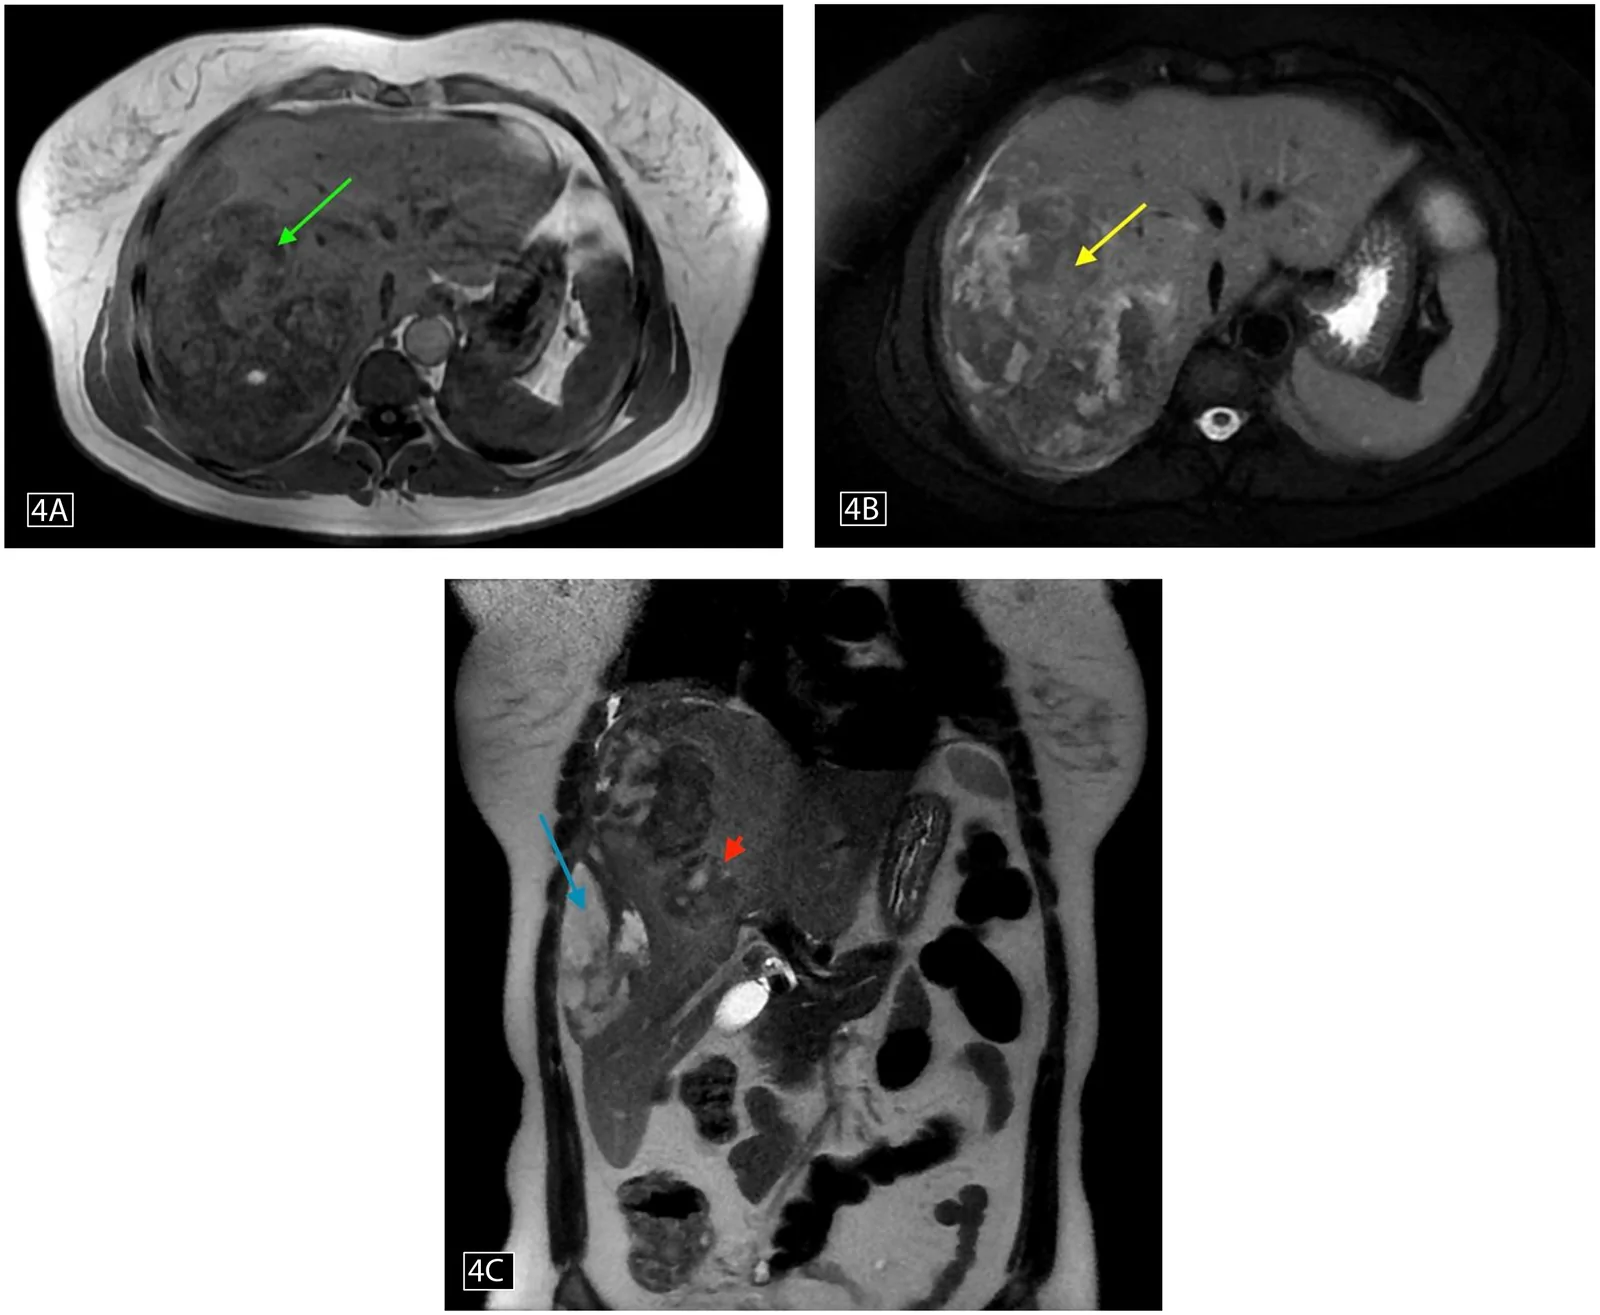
MRI appearance of multiple intrahepatic and subcapsular lesions. (A–C) MRI showing multiple intrahepatic lesions and an associated subcapsular hematoma. The largest lesion demonstrates heterogeneous predominantly isointense signal on T1-weighted imaging (green arrow) (A) and heterogeneous hyperintense signal on T2-weighted imaging (yellow arrow) (B). The coronal image (C) confirms its subcapsular component and demonstrates a separate smaller lesion in segment V (red arrowhead) with similar hemorrhagic imaging features, thereby confirming the multifocal nature of the disease.
